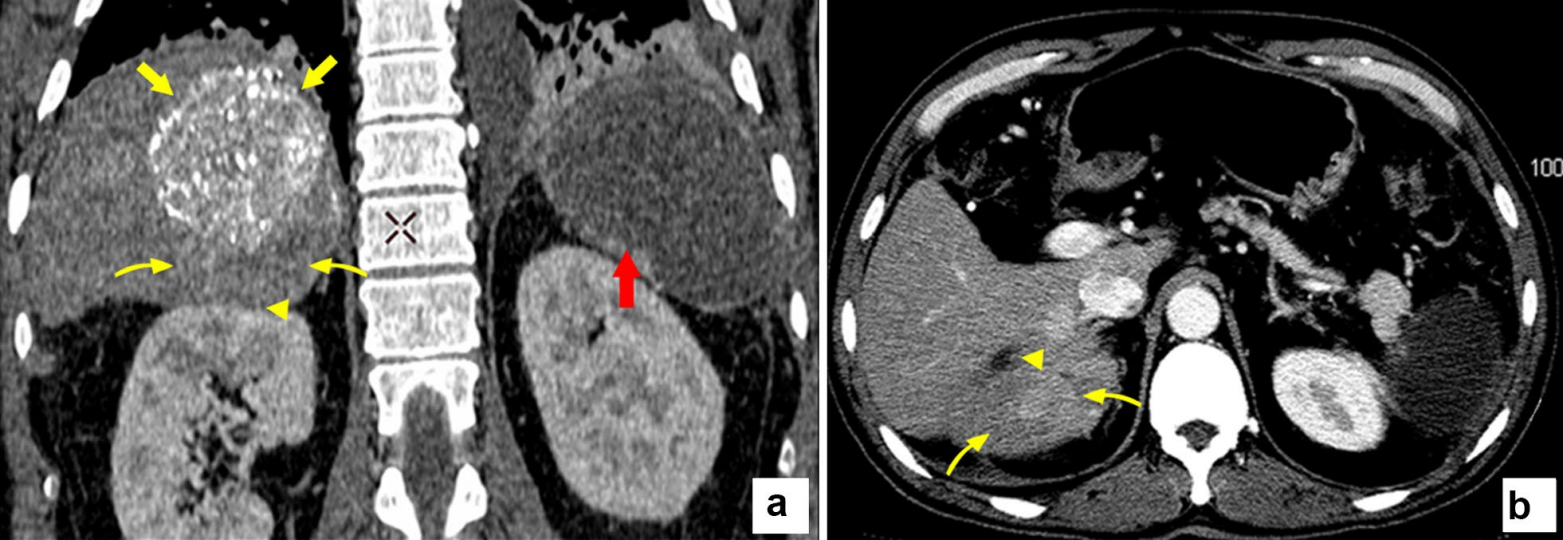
The follow-up CT images after transarterial chemoembolization (TACE). The coronal a and axil b CT images show the tumor with inhomogeneous deposition of lipiodol, the compact upper portion (*yellow arrow*) and the lower portion (*yellow curved arrow*) without lipiodol deposition which was still enhanced in the arterial phase. The protruded tumor was located adjacent to the upper polar of the right kidney (*yellow arrowhead*). The spleen was inactive after partial embolization (*red arrow*)
